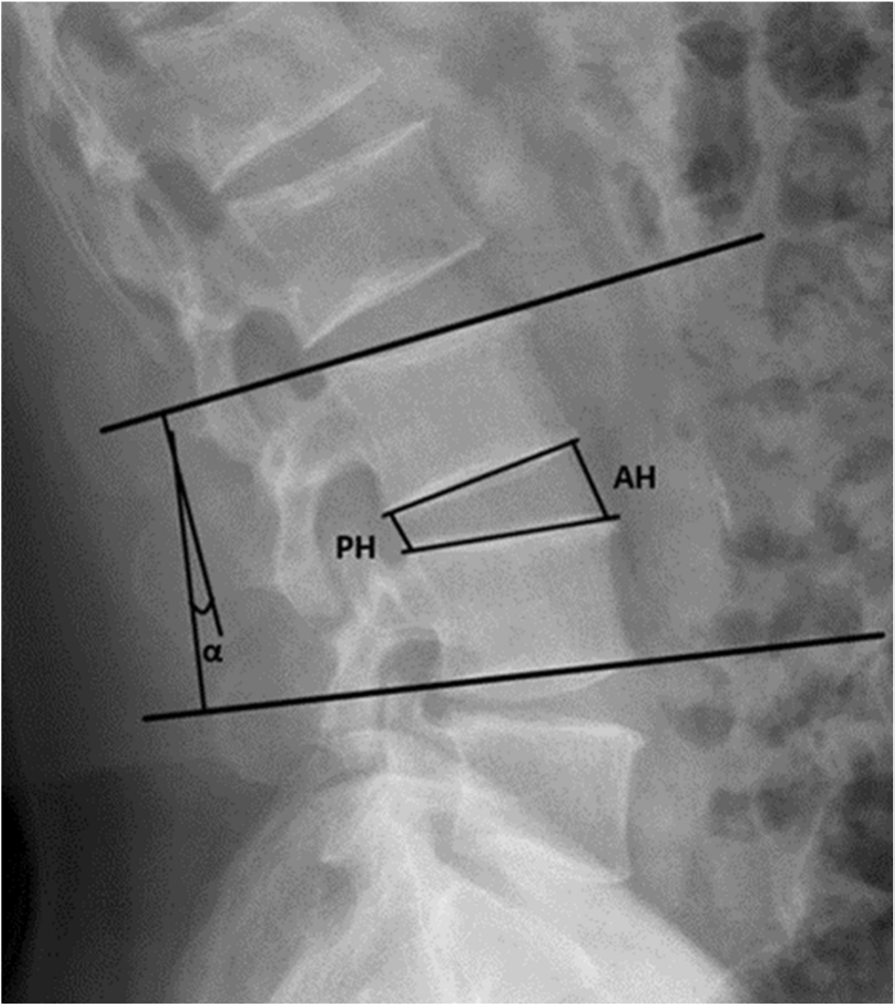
Intervertebral space height (ISH) The height of the intervertebral space is expressed by the mean value of the anterior height (AH) and the posterior height (PH) of the vertebral body on the lateral X-ray of the lumbar spine. Range of motion (ROM) The lumbar vertebral range of motion was indicated by the Cobb angle α of the operative segment and the adjacent segment. The Cobb angle α of the lumbar vertebrae was measured in the lateral, hyperextension, and hyperflexion positions. For the L5-S1 ROM, the upper endplate of the L5 vertebral body and the upper endplate of the sacrum were selected for measurement
